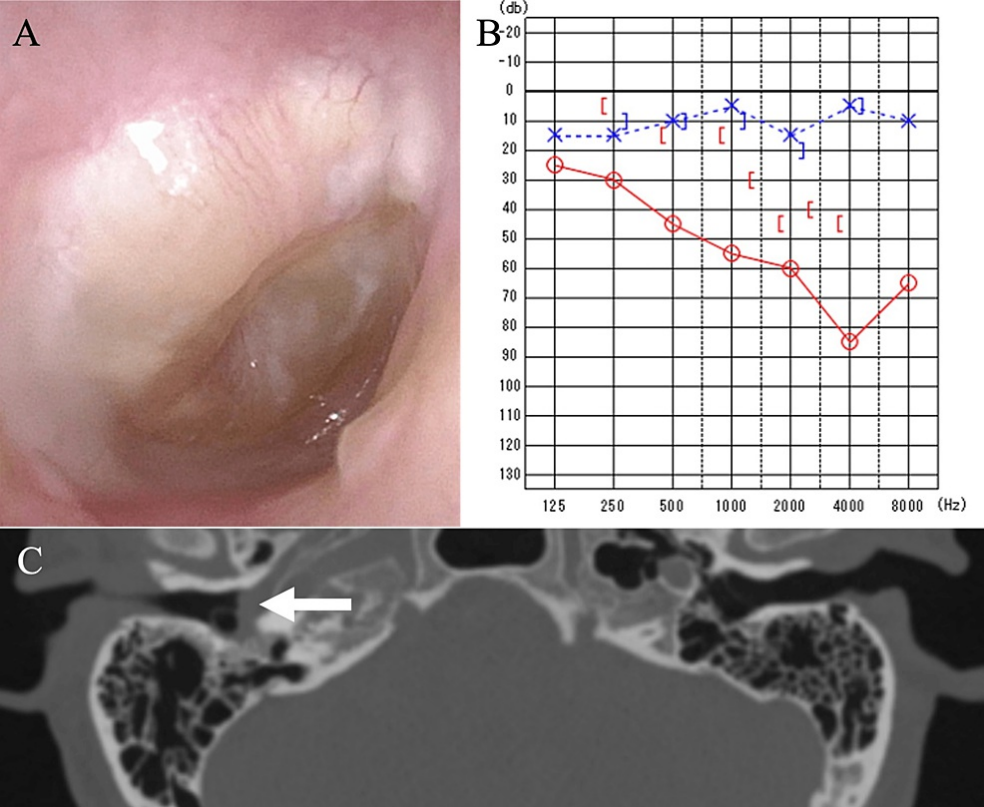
Otoscopic image, pure-tone audiogram, and computed tomography (A) Otoscopic image of the right ear at the first visit shows a bulging tympanic membrane with opaque fluid in the tympanic cavity. (B) Pure-tone audiogram at the first visit reveals a mixed hearing loss of 55 dB in the right ear (red line). (C) Axial computed tomography shows opacification of the right tympanic cavity without bony destruction (arrow).
